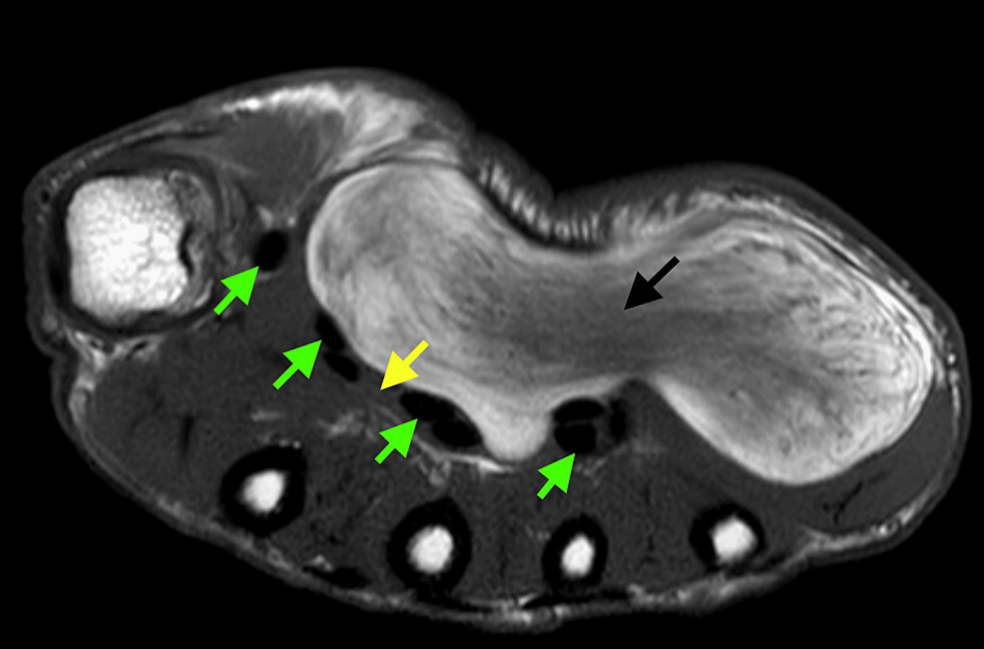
T2-weighted MRI image showing the anatomical relation of the tumor with deep structures of the hand. Black arrow, lipoma; yellow arrow, median nerve; green arrows, flexor tendons. MRI, magnetic resonance imaging
